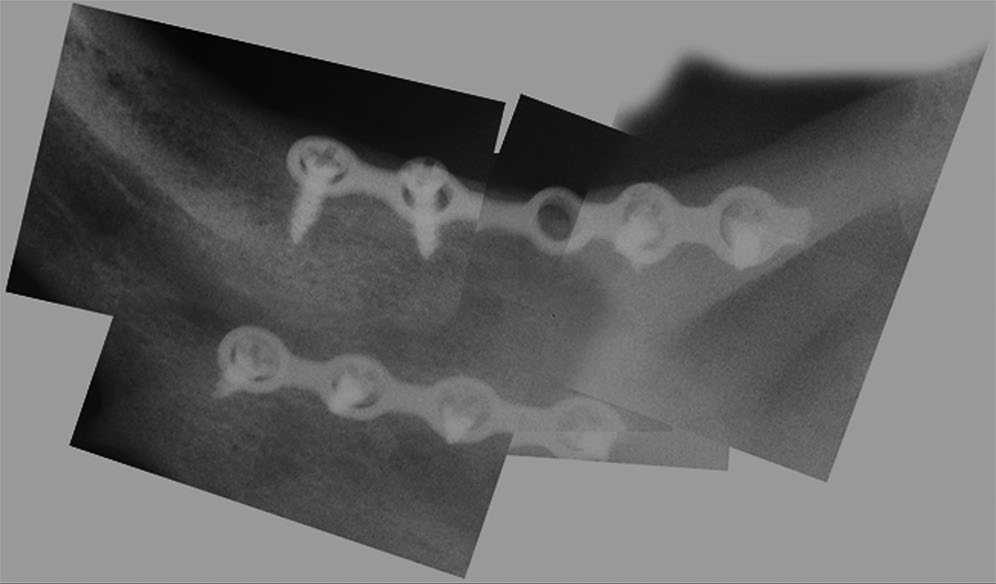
The collage of the periapical radiographs showing the osteosynthesis on the left angle of the mandible of the unidentified human remains.
